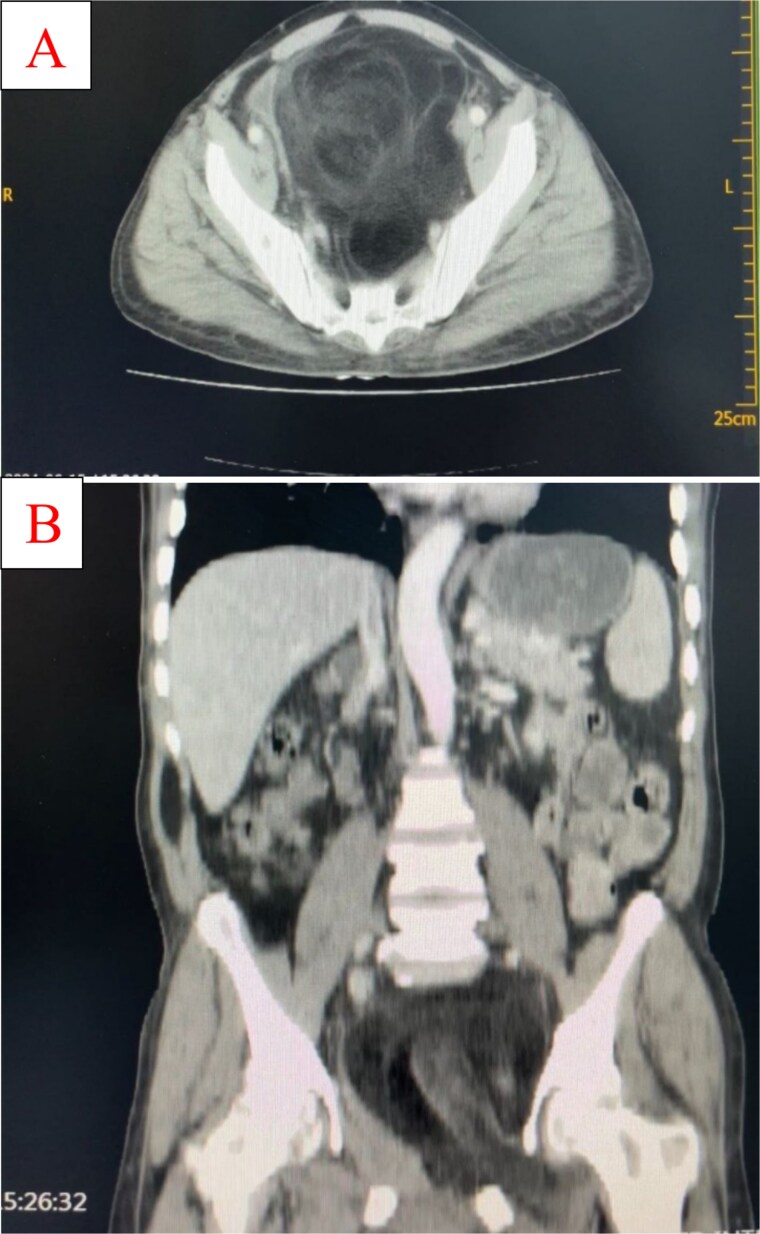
Coronal (A) and sagittal (B) CT images demonstrating a large retroperitoneal fatty mass with central soft tissue density. The mass extends through the left obturator and right greater sciatic foramina, displacing the bladder anteriorly and pelvic organs laterally.
